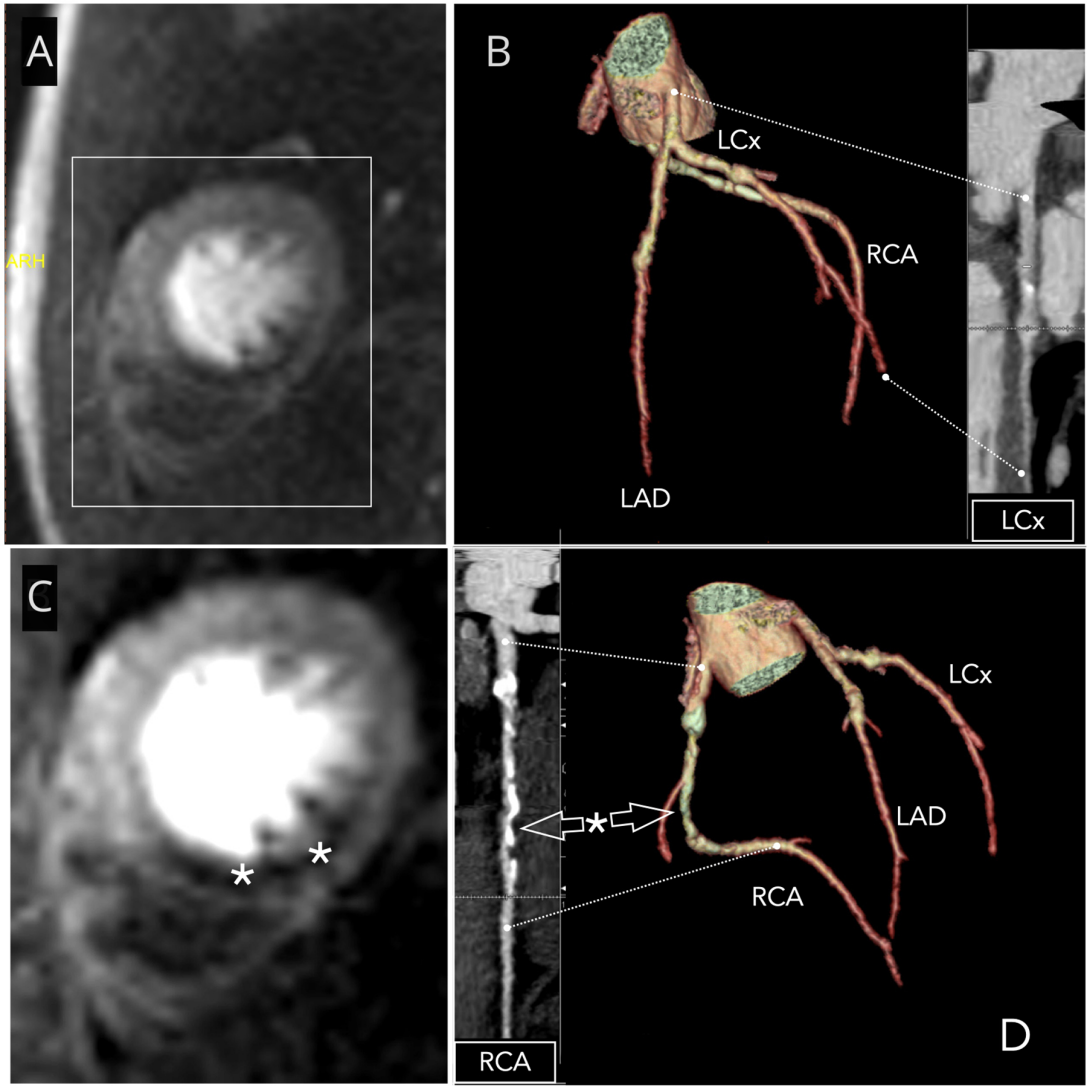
Coronary CT and stress MRI*.*
